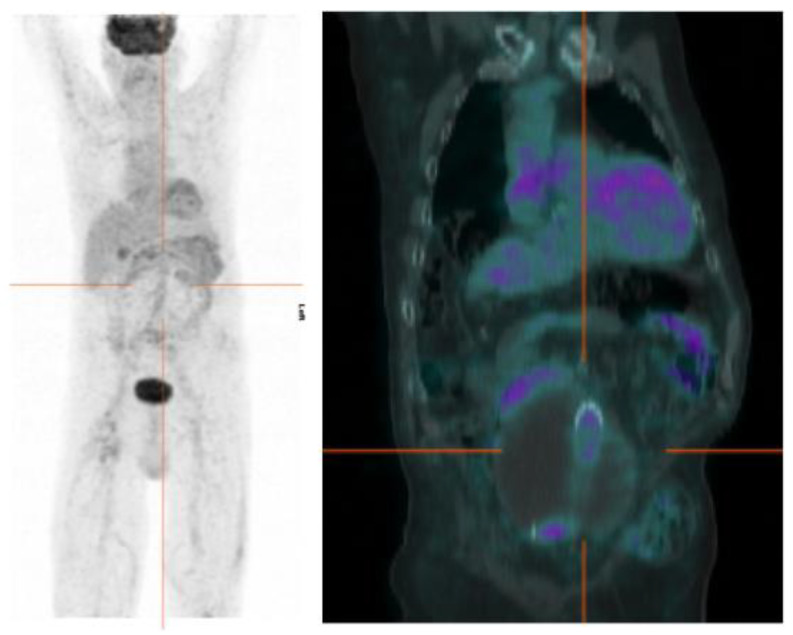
An example of negative [^18^F]FDG PET/CT scan in a patient with suspected infection of abdominal graft implanted 2 years before for the exclusion of a large aneurysm. The images show mild (SUVmax 2.4), homogeneous uptake along the whole tract of the prosthesis without any focal uptake.
